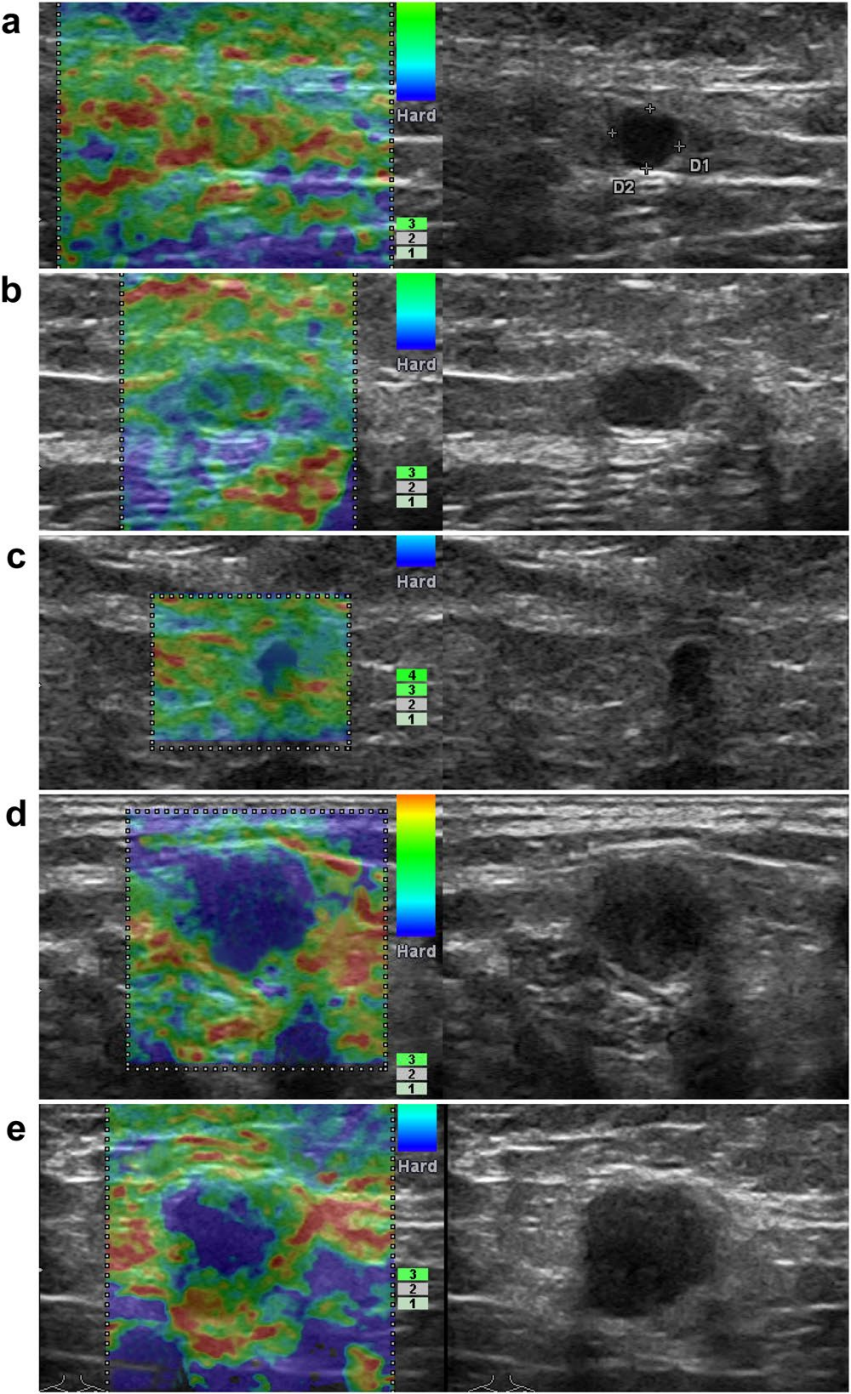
Grey scale-RTE dual-mode ultrasonogram of Pattern II ALNs. Images present the general appearance of lesions for RTE scores of (a) 1, (b) 2, (C) 3, (d) 4, and (e) 5 of the lymph nodes without hila: (a) Green portion occupying almost all of the cortex; (b) Blue portion occupying less than 50% of the cortex; (c) Blue portion occupying more than 50% of the cortex, with a scattered green/red portion; (d) Blue portion occupying almost all of the cortex; (e) Blue portion occupying almost all of the cortex, with a green/red ring on the edge of the node.
